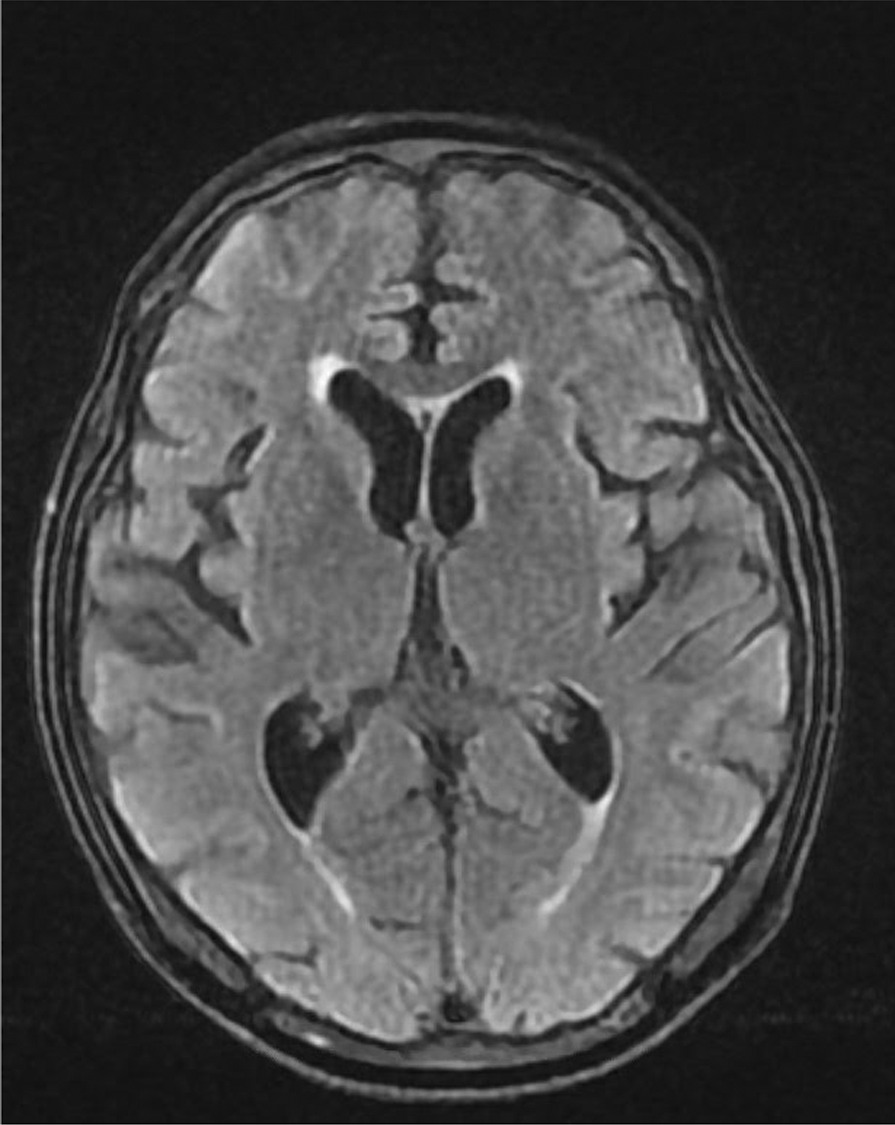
Magnetic resonance imaging (MRI) of the brain combined shows moderate diffuse cerebral and cerebellar atrophy without evidence for intraparenchymal brain metastasis
